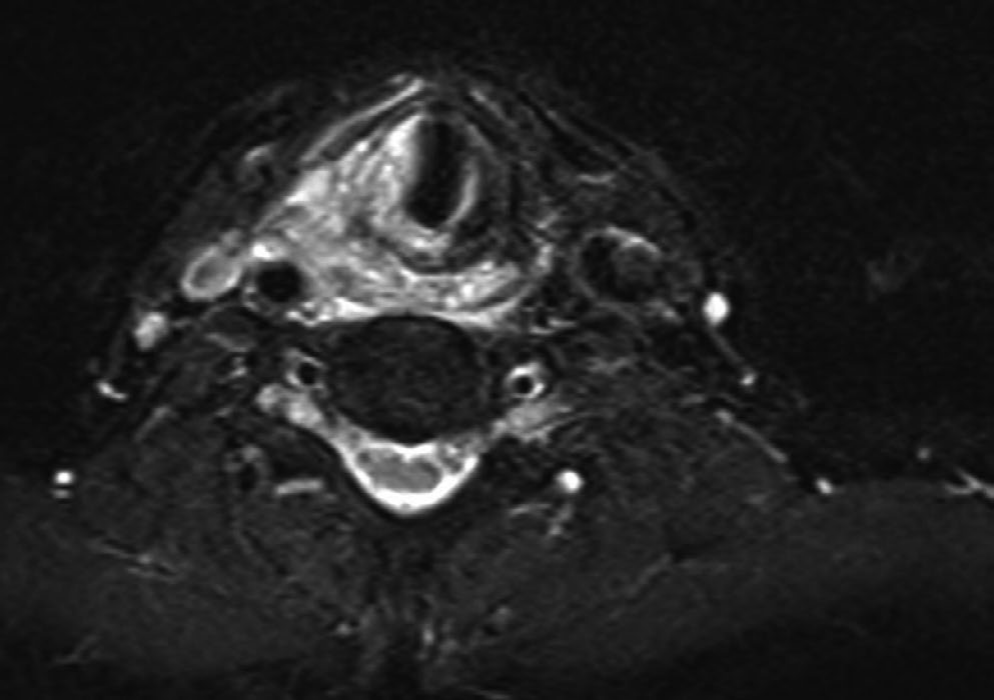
T2 weighted axial MRI image of tumor invading right thyroid cartilage and encasing right carotid
